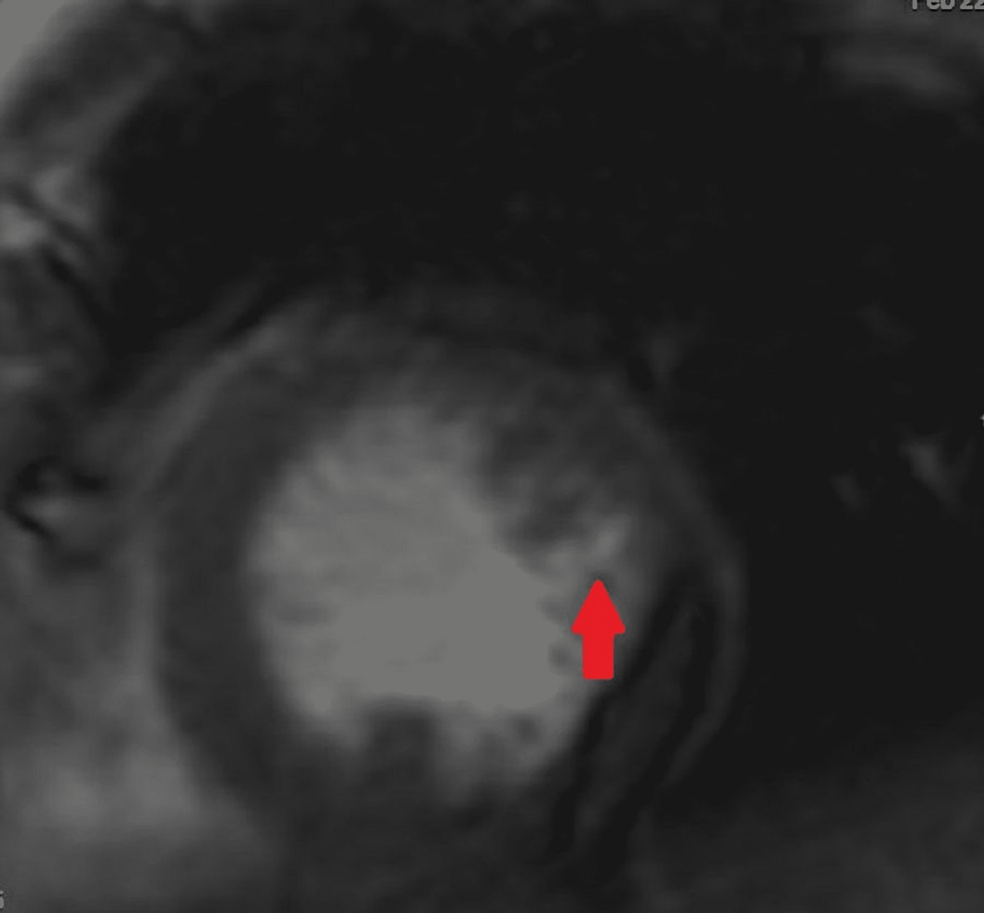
Cardiac MRI demonstrating left ventricular non-compacted myocardium (red arrow)
